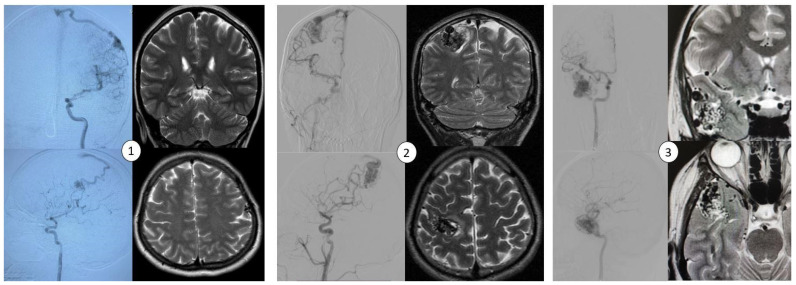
Neuroradiological imaging findings of bAVM lesions, including cerebral angiograms in both anterior and lateral projections and MRI scans (T2-weighted sequences) in the axial and coronal planes. The images correspond to the three patients described in the study: (1) AVM1—located in the left frontal lobe, (2) AVM2—located in the right parietal lobe, and (3) AVM3—located in the right temporal lobe.
